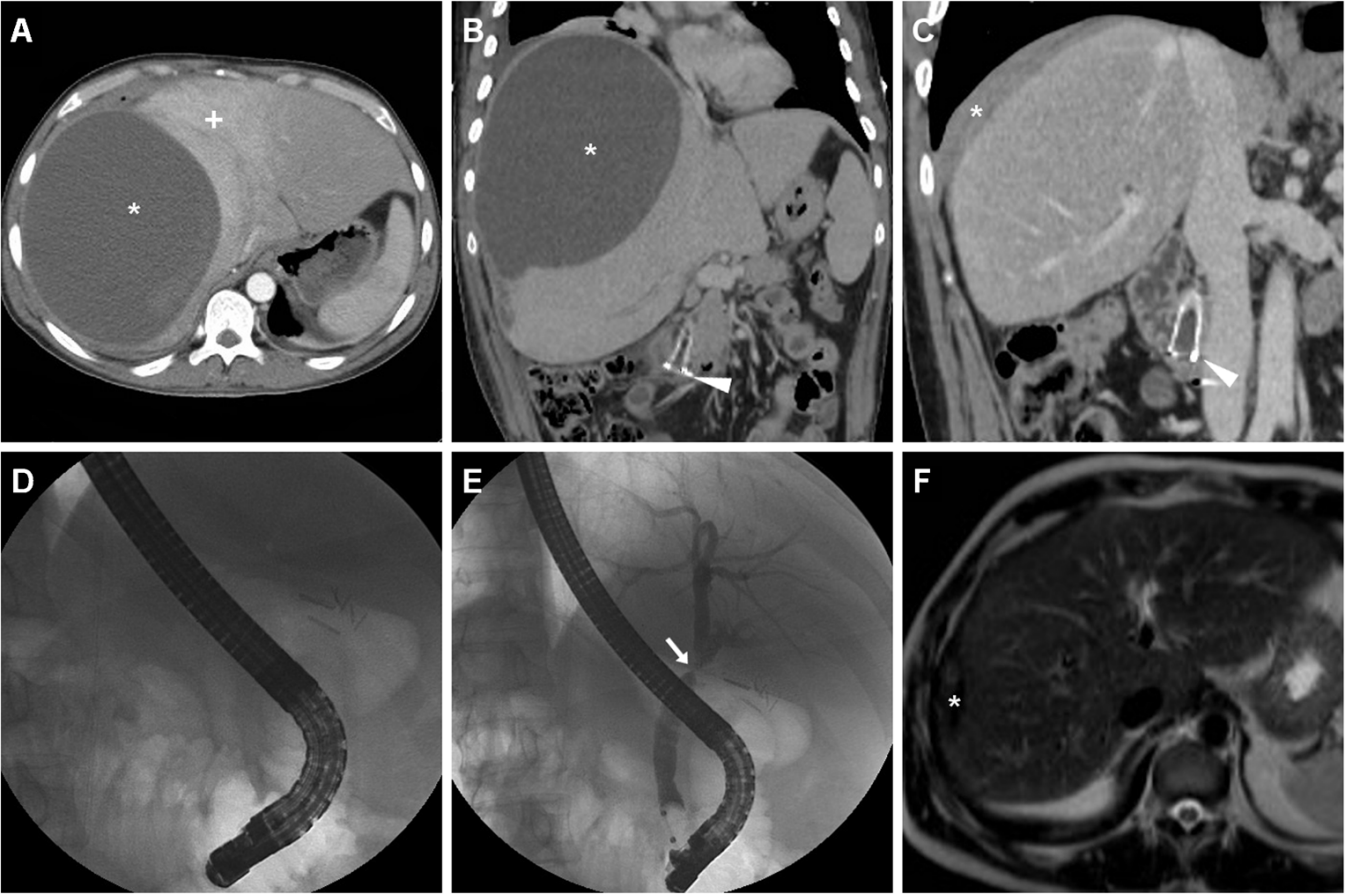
One month after evacuation of the haemoperitoneum and subcapsular hepatic haematoma, the same patient in Fig. 10 suffered form right-sided thoraco-abdominal pain and fever. Arterial- (a) and venous-phase (b) CT showed a huge, fluid-attenuating (15 HU) subphrenic collection (*) with inflammatory enhancement of the surrounding, compressed liver parenchyma (+) and thin peripheral enhancement, consistent with an abscess. Ipsilateral pleural effusion and basal lung atelectasis were associated. Percutaneous drainage yielded 3 l of stinking pus. Note the correctly positioned metallic CBD stent (*arrowheads*), and clinical, laboratory and imaging (* in c) resolution was obtained. During endoscopic replacement, the biliary stent was not found anymore (d), displaced and probably lost with stools. Endoscopic cholangiogram (e) confirmed persistent iatrogenic biliary stricture (*arrow*). Follow-up MRI (T2-weighted image in f) showed resolved subphrenic abscess with low signal intensity consistent with fibrosis (*)
